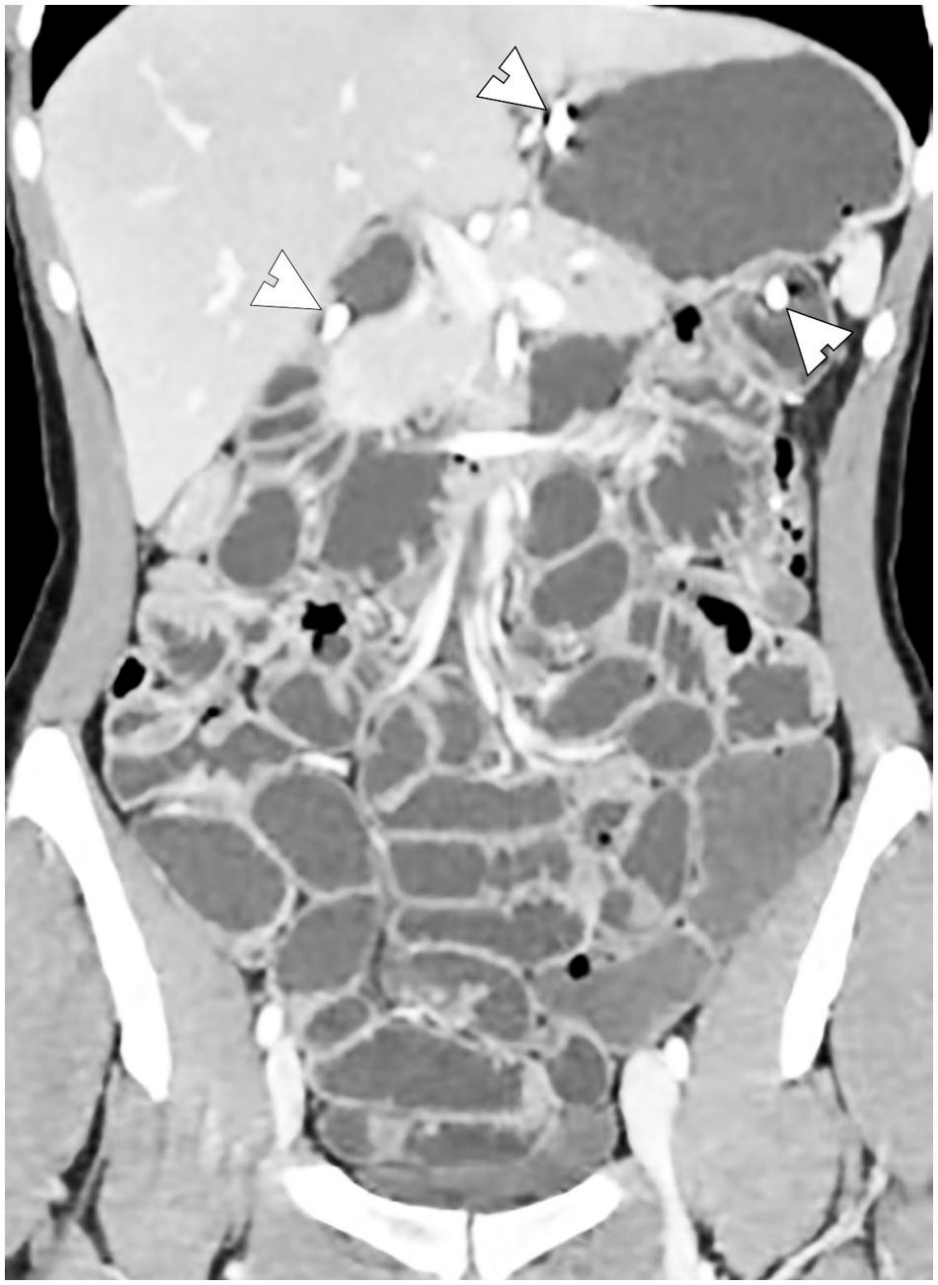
Example of good small bowel distension in CT-Enteroclysis: coronal CT image, acquired after administration of the contrast agent (methylcellulose) through nasojejunal tube and iodinated contrast medium injection, shows adequate homogeneous distension of the whole small bowel. Nasojejunal tube is clearly evident (arrowheads).
